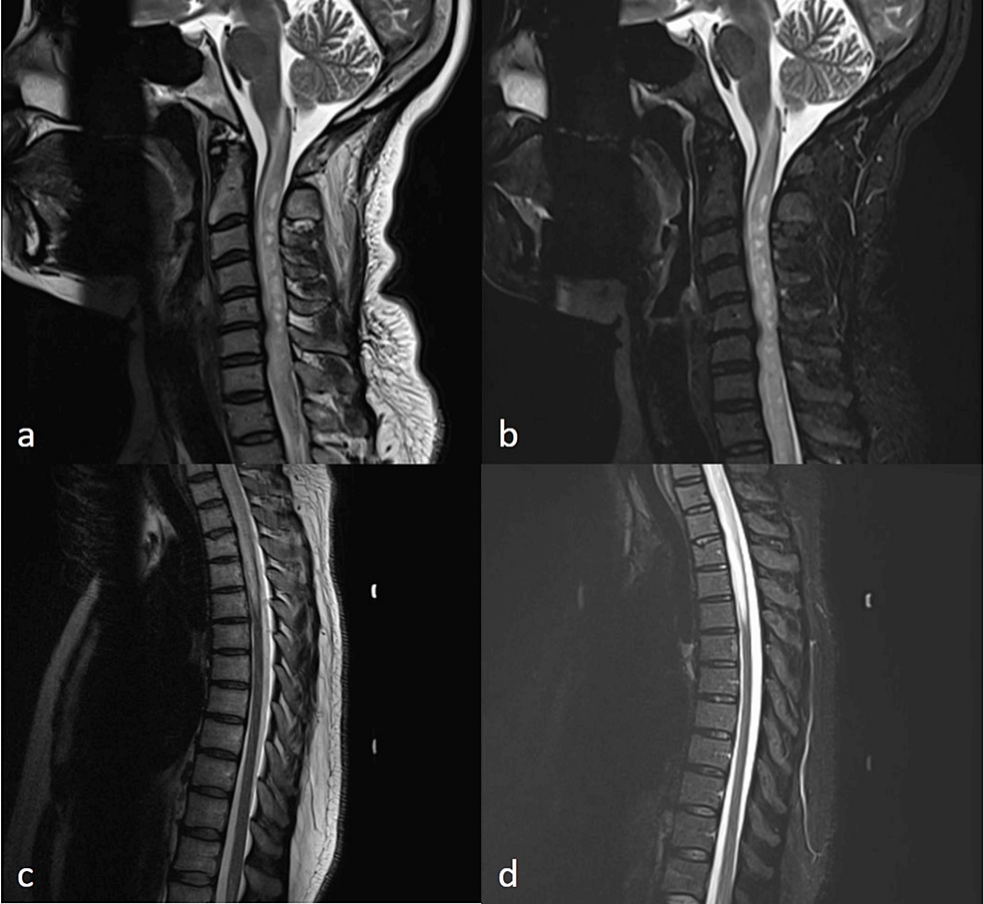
Sagittal T2 (a and c) and T2 short-tau inversion recovery (b and d) weighted magnetic resonance images of the cervical (a and b) and thoracic (c and d) spine. Cervical images show extensive cervical spinal cord signal abnormality with thick enhancement of the posterior aspect of the spinal cord and/or adjacent dura. At C5-6, there is degenerative disc disease with disc bulge spurs and cord flattening. At C6-7, there is degenerative disc disease with mild disc bulge and mild cord flattening. Thoracic images show diffuse signal abnormality in the upper thoracic cord to the T5-6 level with thick posterior enhancement to the T2-3 level.
